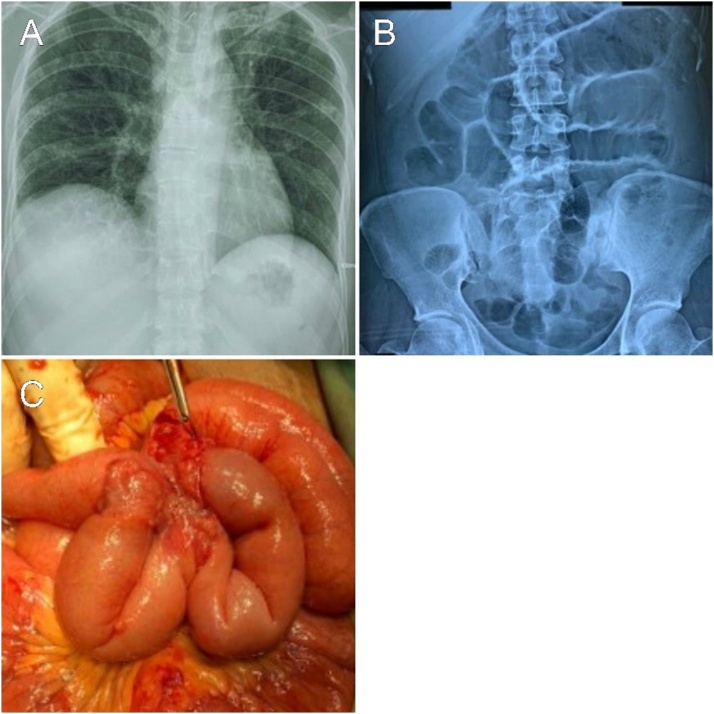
A) Chest X-ray showed atypical bronchopneumonia, B) Abdominal X-ray showed small bowel obstruction suspected ileus, and C) Intraoperative finding of multiple intestinal strictures and stenosis.
